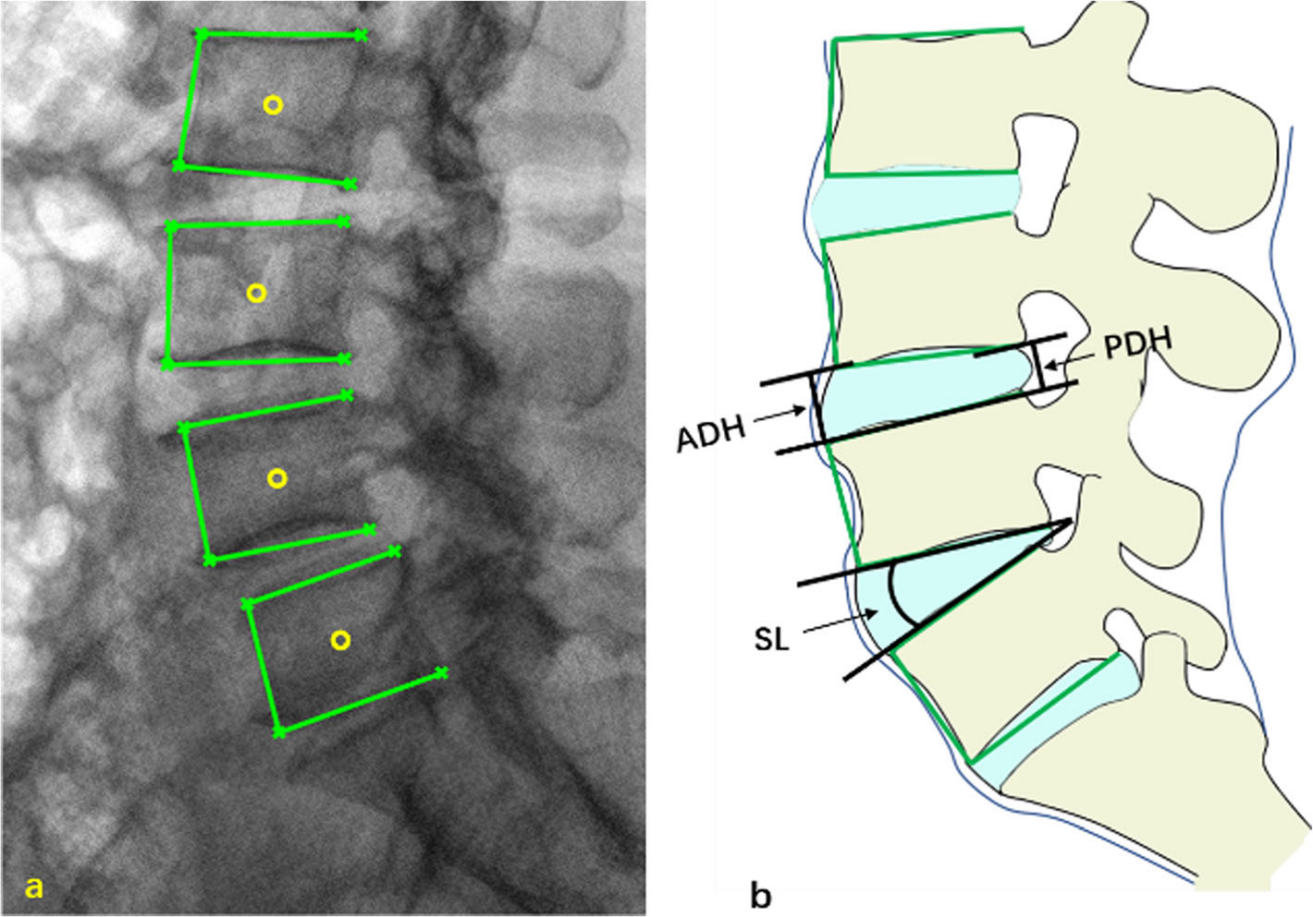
Parameter Measurement: a. recognition of vertebral margins via X-ray films; b. calculation of AHD, PDH and SL parameters
